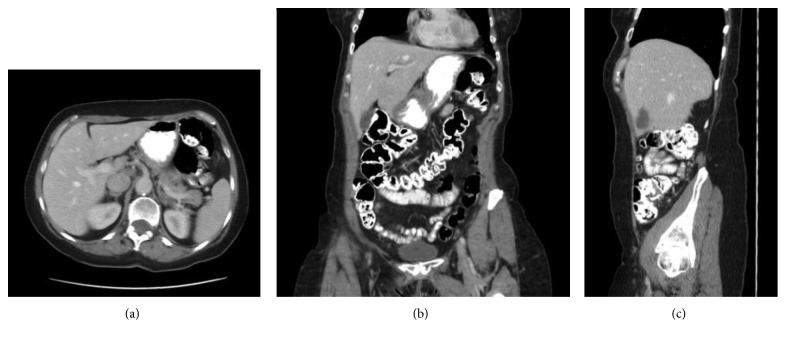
(a) Axial, (b) coronal, and (c) sagittal images from the patient's 2015 CT abdomen and pelvis showing no colon anterior to the liver.
